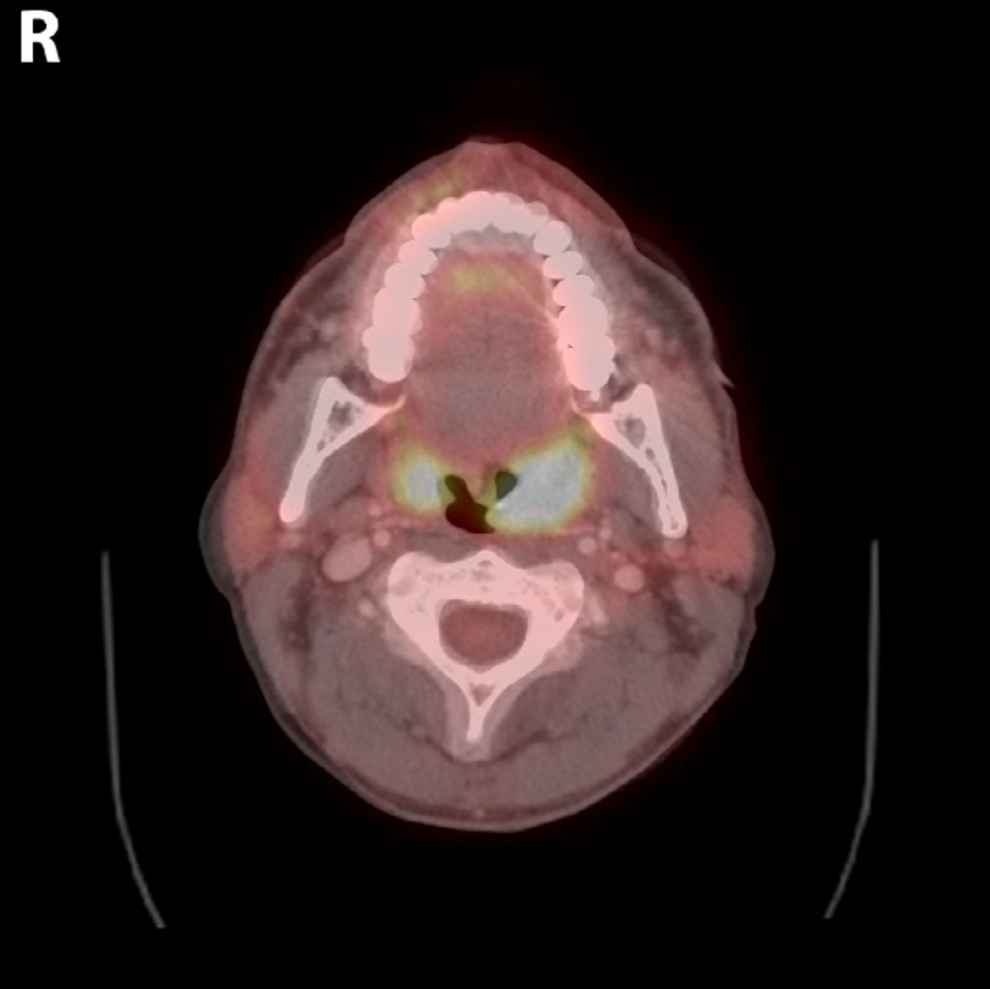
Axial view of whole body FDG‐PET/computed tomographic (CT) layered at 55% opacity over CT scan, allowing for improved visualization of the tumor margins in the oropharynx
